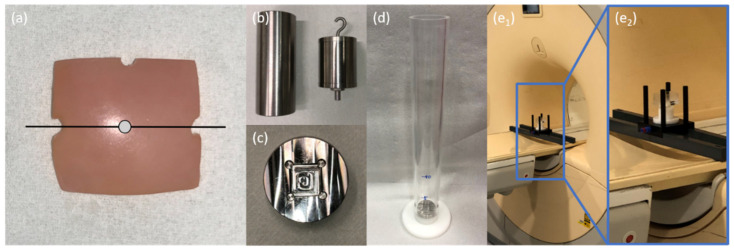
Details of cartilage sample preparation and subsequent injurious impaction loading. (a) Top view of a representative osteochondral sample. Notches at the 3 and 9 o’clock positions indicate the mid-sagittal plane (black line), while the sample centre point is defined as the intersection of this mid-sagittal plane and its perpendicular along the notch at the 12 o’clock position (grey dot). (b) Weights of 1000 g (left) and 500 g (right) equipped with hook and 5 mm-tip to induce standardized injurious impaction. (c) Metallic sample plate with flat recess to fix cartilage samples during impaction by preventing lateral displacement. Seeming distortions are secondary to the milling process and room lights. (d) Cylindrical drop-tower device with metallic sample plate and indications of height. Impaction energy levels were regulated by adjustment of weight and height. Details of the assembled measurement framework at variable magnifications (blue boxes) within the scanner’s bore. The framework included support beams mounted on the MRI table (e_1_) and a transparent sample box (containing the cartilage samples, (e_2_)) for reproducible positioning of sample and coil (not shown).
